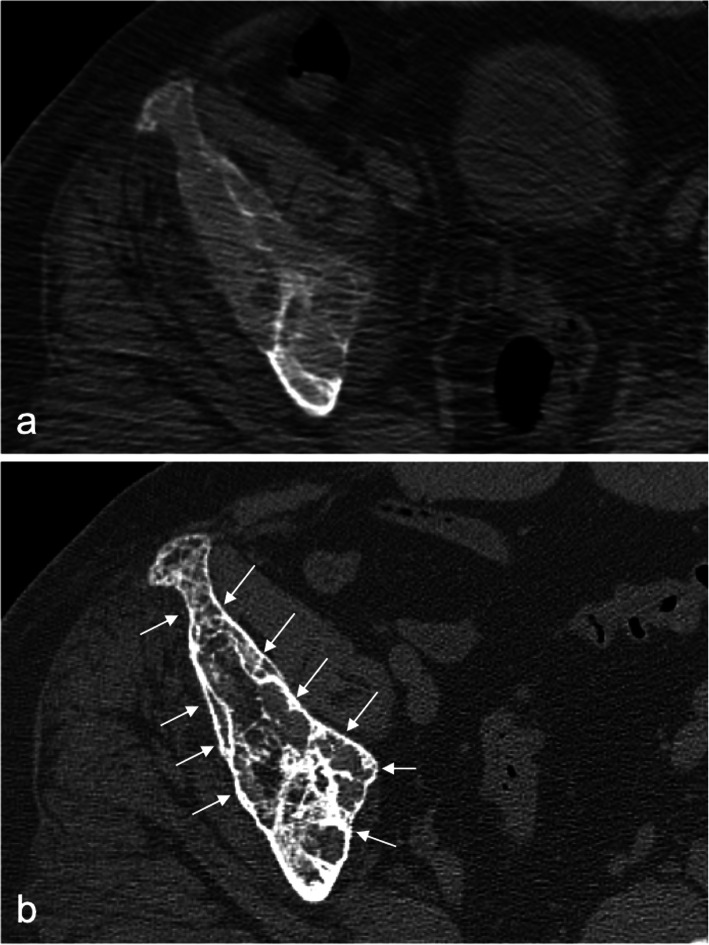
Initial single-row detector computed tomography (CT) of the pelvis showing extensive lytic lesion, affecting the entire right iliac bone, with destruction of bone cortices and the presence of some normal bony streaks. This lesion is spontaneously hyperdense (130 UH). There is also diffuse bone enlargement without the coarsened trabecular pattern found in Paget disease (a). Ten months after parathyroidectomy (b), CT shows advanced healing of the lytic lesion of the right iliac bone with complete reconstruction of bone cortices (small arrows) and formation of intra medullary, thickened bone septa
